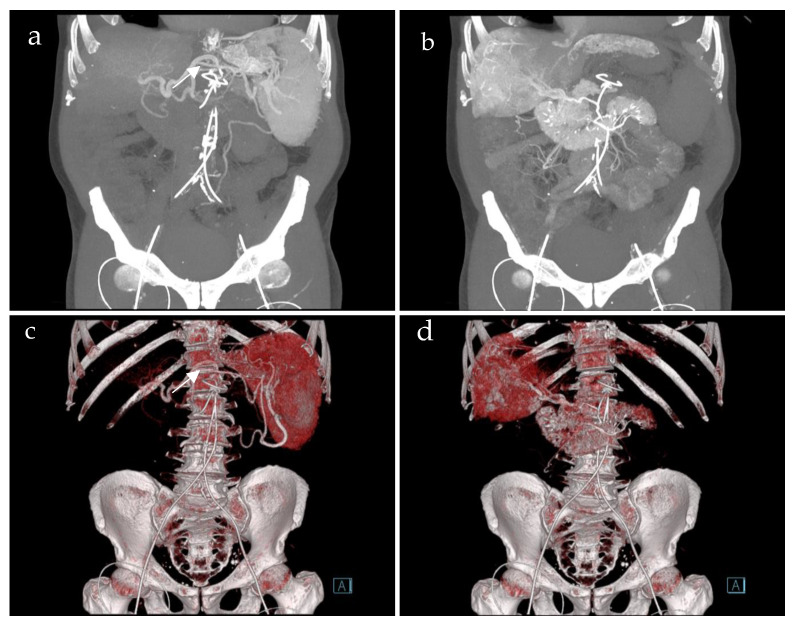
Coronal images of computed tomography spleno-mesenterico-portography of a 72-year old patient with portal hypertension showing the catheters in the splenic artery (a,c) (white arrow) and the superior mesenteric artery (b,d), both via the groin. Maximum intensity projection (a,b) and 3D models (c,d). Computed tomography splenoportography (a,c) detects the contrasted enlarged spleen, the splenic vein and extrahepatic portal vein. Computed tomography mesenterico-portography (b,d) shows the contrasted duodenum, liver and extrahepatic portal vein.
